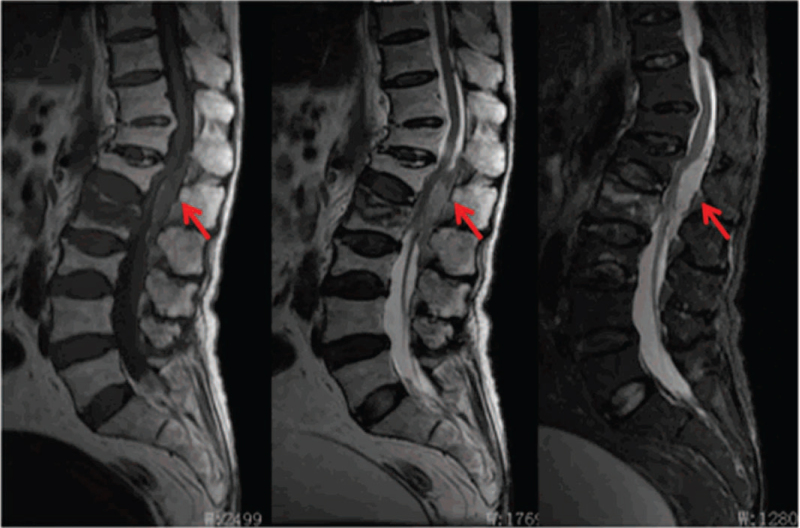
The preoperative MRI performed on admission revealed L2 fresh vertebral fractures accompanying a well-defined ovoid lesion in the dorsal epidural region at T12-L3 level that caused compression of the spinal cord. The lesion was isointense signals of the spinal cord with a thin hyperintense signal at the peripheral rim on the T1-weighted images, and mixed signal intensity on the T2-weighted images (indicated by red arrow). MRI = magnetic resonance imaging.
